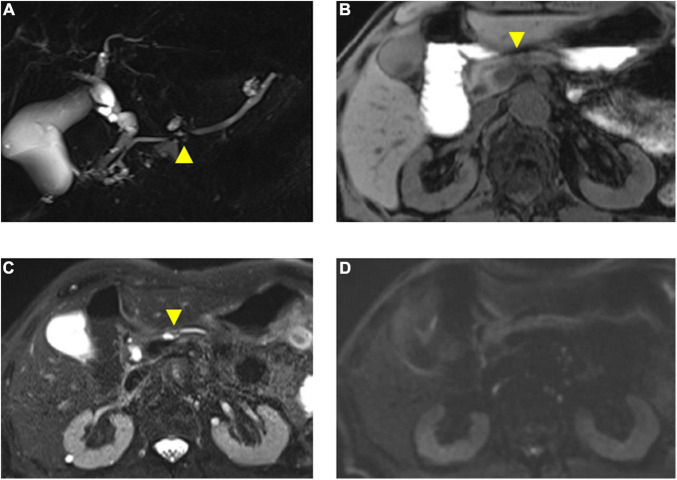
Magnetic resonance imaging findings of the presented case. (A) Magnetic resonance cholangiopancreatography (MRCP) shows disruption of the main pancreatic duct (MPD) (arrowhead) and a nearby cyst. (B) T1-weighted fat saturation MRI shows necking of pancreas parenchyma (arrowhead) with weak low-intensity change in a pancreatic body. (C) T2-weighted MRI shows dilated pancreatic duct and nearby cyst (arrowhead). (D) Diffusion-weighted MRI (*b* = 1,000) shows no sign of malignancy.
